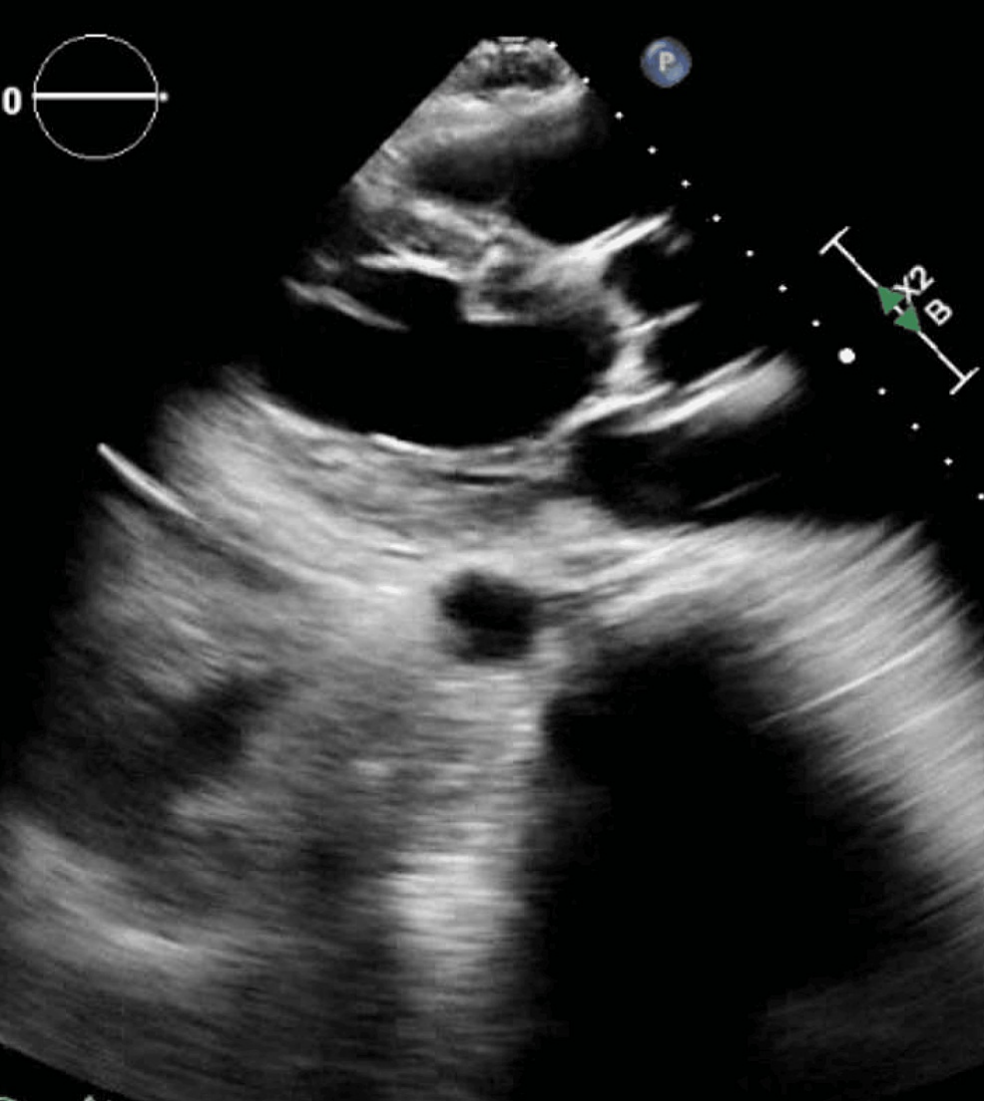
Transthoracic echocardiogram which did not show valvular vegetations.
